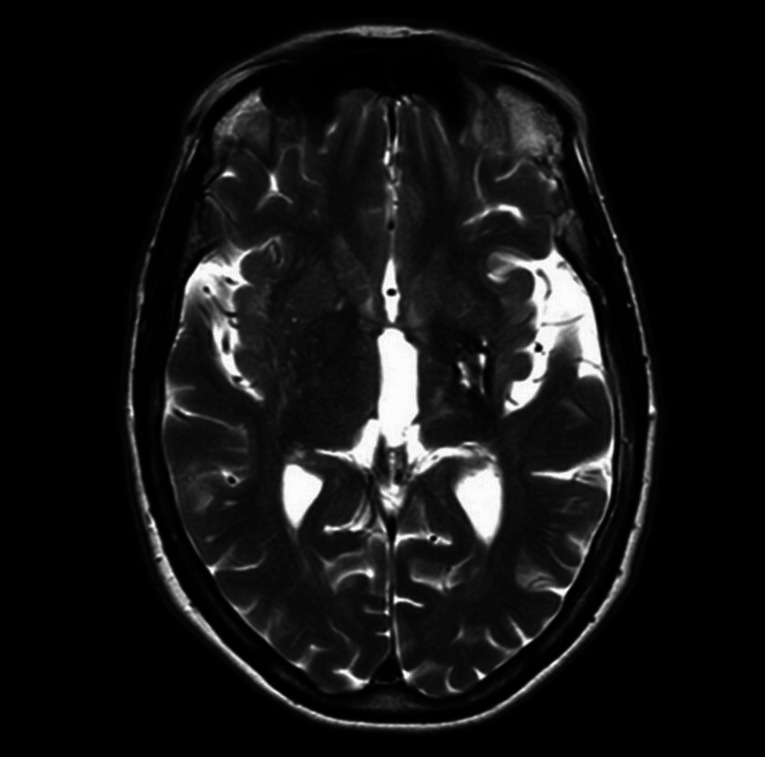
Axial magnetic resonance image showing poststroke changes in the left and right hemisphere.
